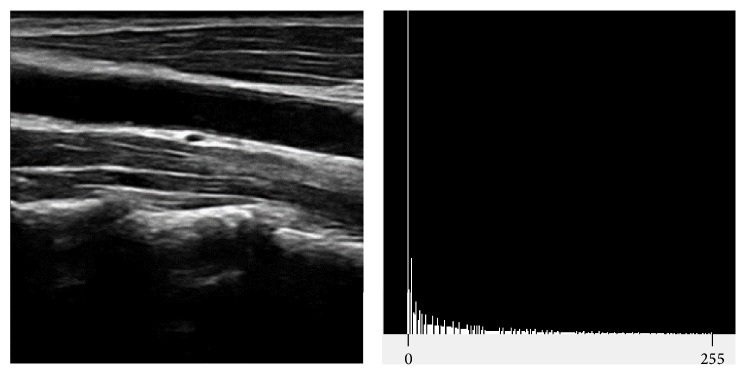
Cervical vertebrae sonography and histogram.
